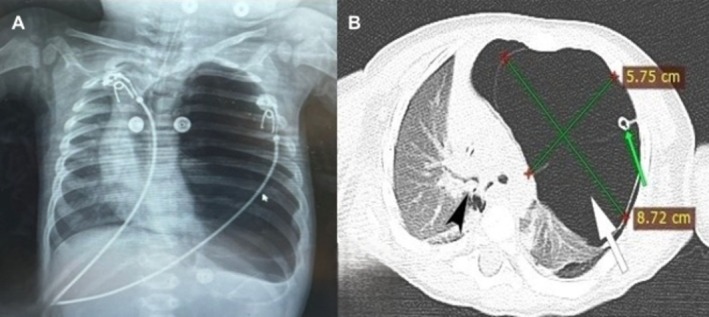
(A) Chest radiograph obtained at the referring hospital demonstrating marked hyperlucency of the left hemithorax with rightward mediastinal shift, initially interpreted as left‐sided tension pneumothorax. (B) Axial computed tomography (CT) image of the chest showing a large multicystic lesion occupying the left hemithorax consistent with congenital pulmonary airway malformation (white arrow), a foreign body obstructing the right main bronchus with extension into the middle and lower lobar bronchi (black arrow), and a left‐sided thoracic tube (green arrow).
